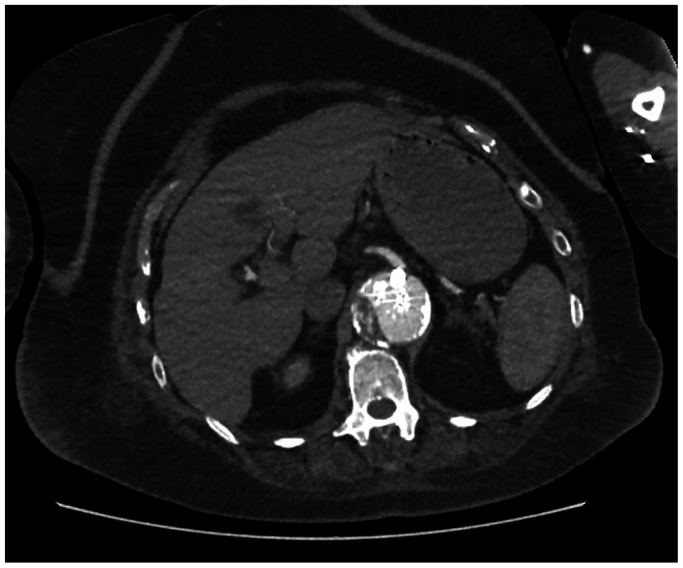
Computed tomography angiography performed after the first-stage branched endovascular aneurysm repair (BEVAR), showing a type III endoleak caused by intentional preservation of the celiac trunk branch.
